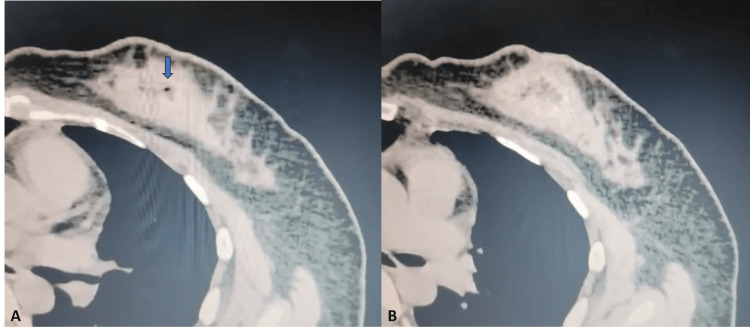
Computed tomography images (case two) (A) and (B) show an irregular soft tissue density mass in the central and inner quadrant of the left breast with central hypodensity and foci of air (blue arrow) giving a spongiform or honeycomb appearance, characteristic of retained surgical sponge (gossypiboma).
